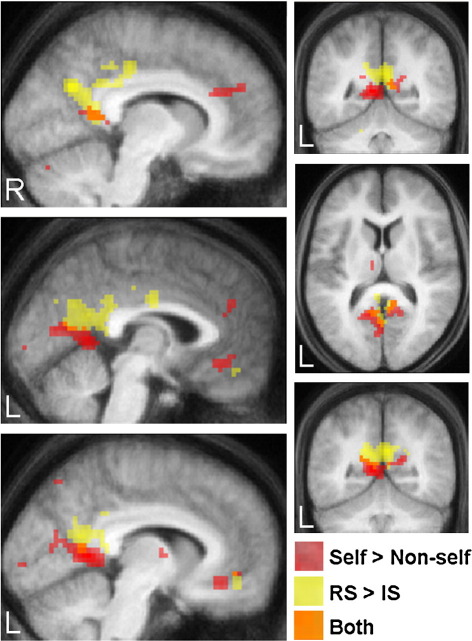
Comparison of brain regions modulated by self and real self. The brain regions preferentially activated when recalling real autobiographical (RS) events compared to imagined autobiographical (IS) events (yellow) are overlaid on the same brain (averaged structural MRI scan of all participants) as regions that were more active for recalling autobiographical events (real and imagined: RS + IS) compared to non-self (film and news, both real and imagined: RF + IF + RN + IN) events (red). Regions that were active in both contrasts are shown in orange. R = right, L = left.
